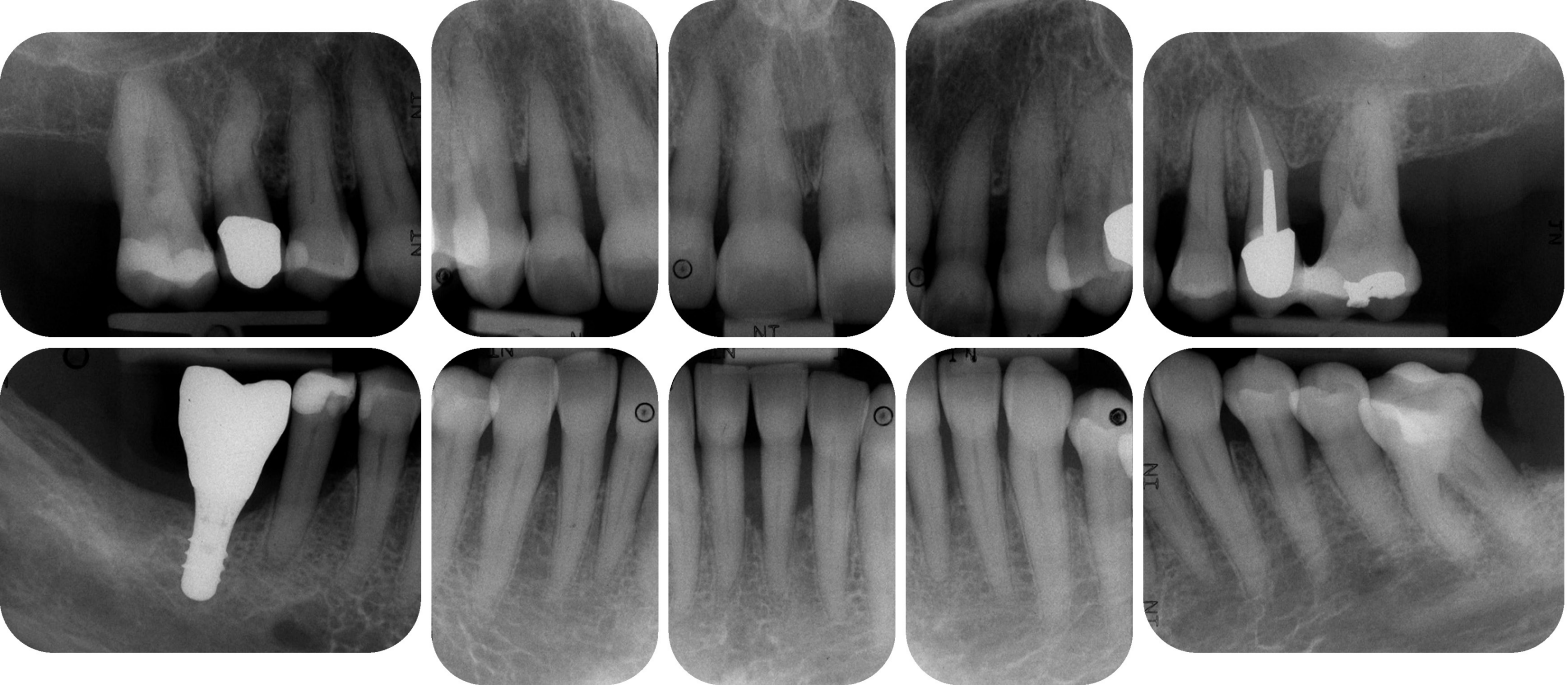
Radiographic view after periodontal therapy, implant placement, and prosthetic restoration indicating healthy periodontal and peri‐implant conditions
